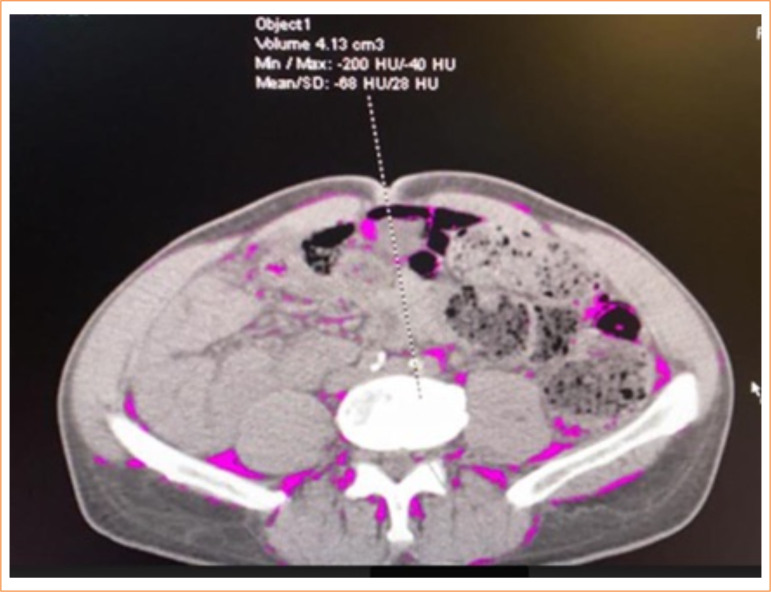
Representative computed tomography scan image at the L3 vertebral level in a patient with rectal adenocarcinoma, showing a low volume of visceral fat, with the pink area indicating the amount of visceral fat in cm^3^.
